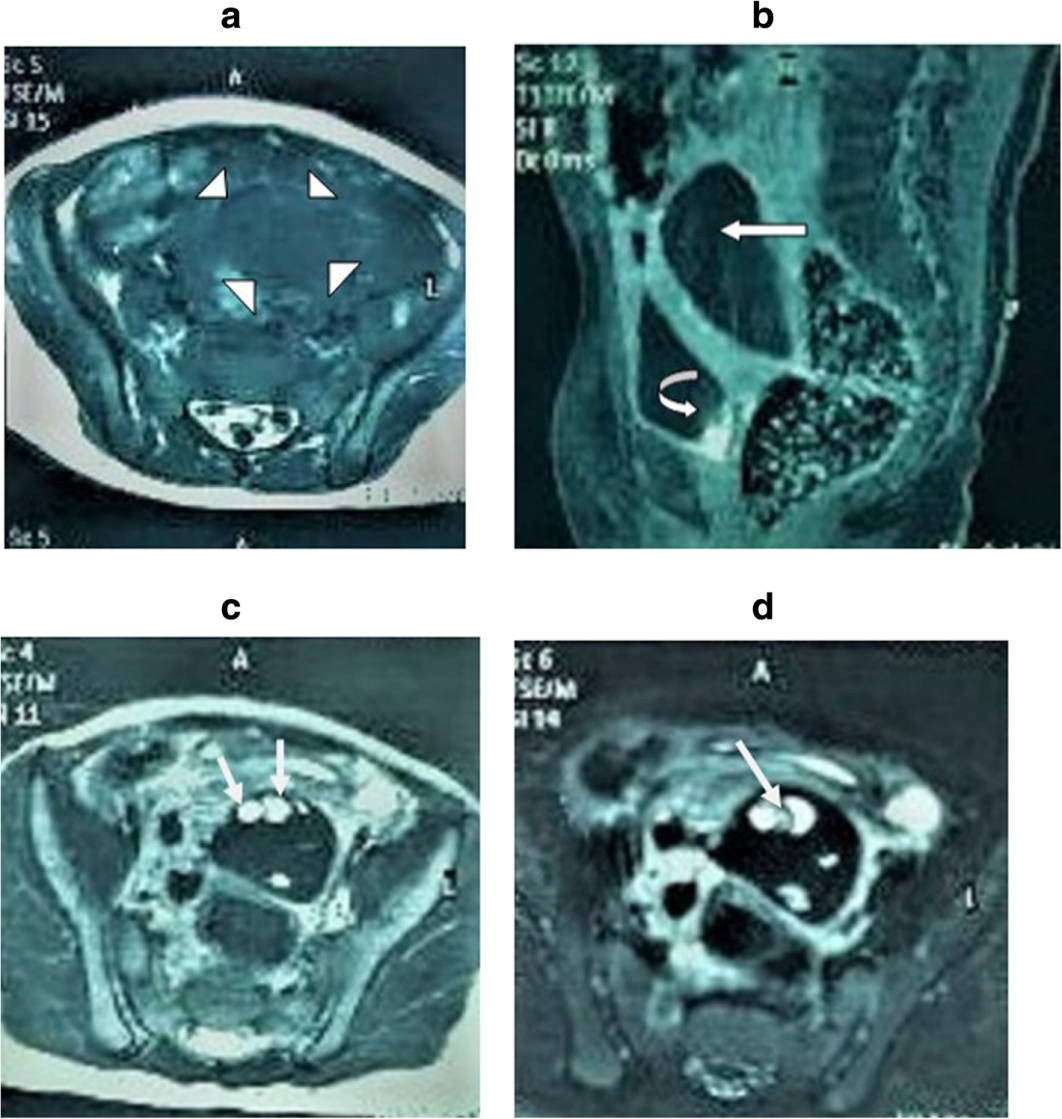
Torsion of the right ovary of a 2-year-old girl with a history of abdominal pain and vomiting. a Axial T1-weighted MR image shows enlarged right ovary (arrowheads). b Sagittal contrast-enhanced fat-suppressed T1-weighted MR image shows no contrast enhancement in the right ovary (arrow). Contrast is seen within the urinary bladder (curved arrow). c Axial T2-weighted MR image shows multiple cystic areas within the enlarged right ovary favoring enlarged follicles (arrows). d Axial T2-weighted fat-suppressed MR image shows hemorrhages of varying stages (arrow). MR magnetic resonance
